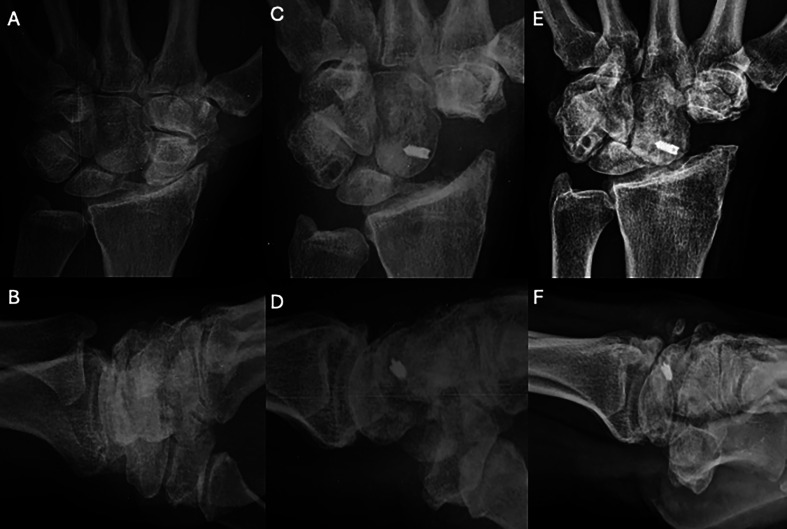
Clinical case of FCR Tenodesis transosseous T (Osseous grip) and Scaphoid excision. A and B. Preoperative wrist X-rays of SLAC stage II (AP and Lateral views). C and D. Postoperative wrist X-rays (AP and Lateral views) after 2 months. E and F. Postoperative wrist X-rays (AP and Lateral views) after 8.5 years. Source: Dr. Emmanouil Apergis.
